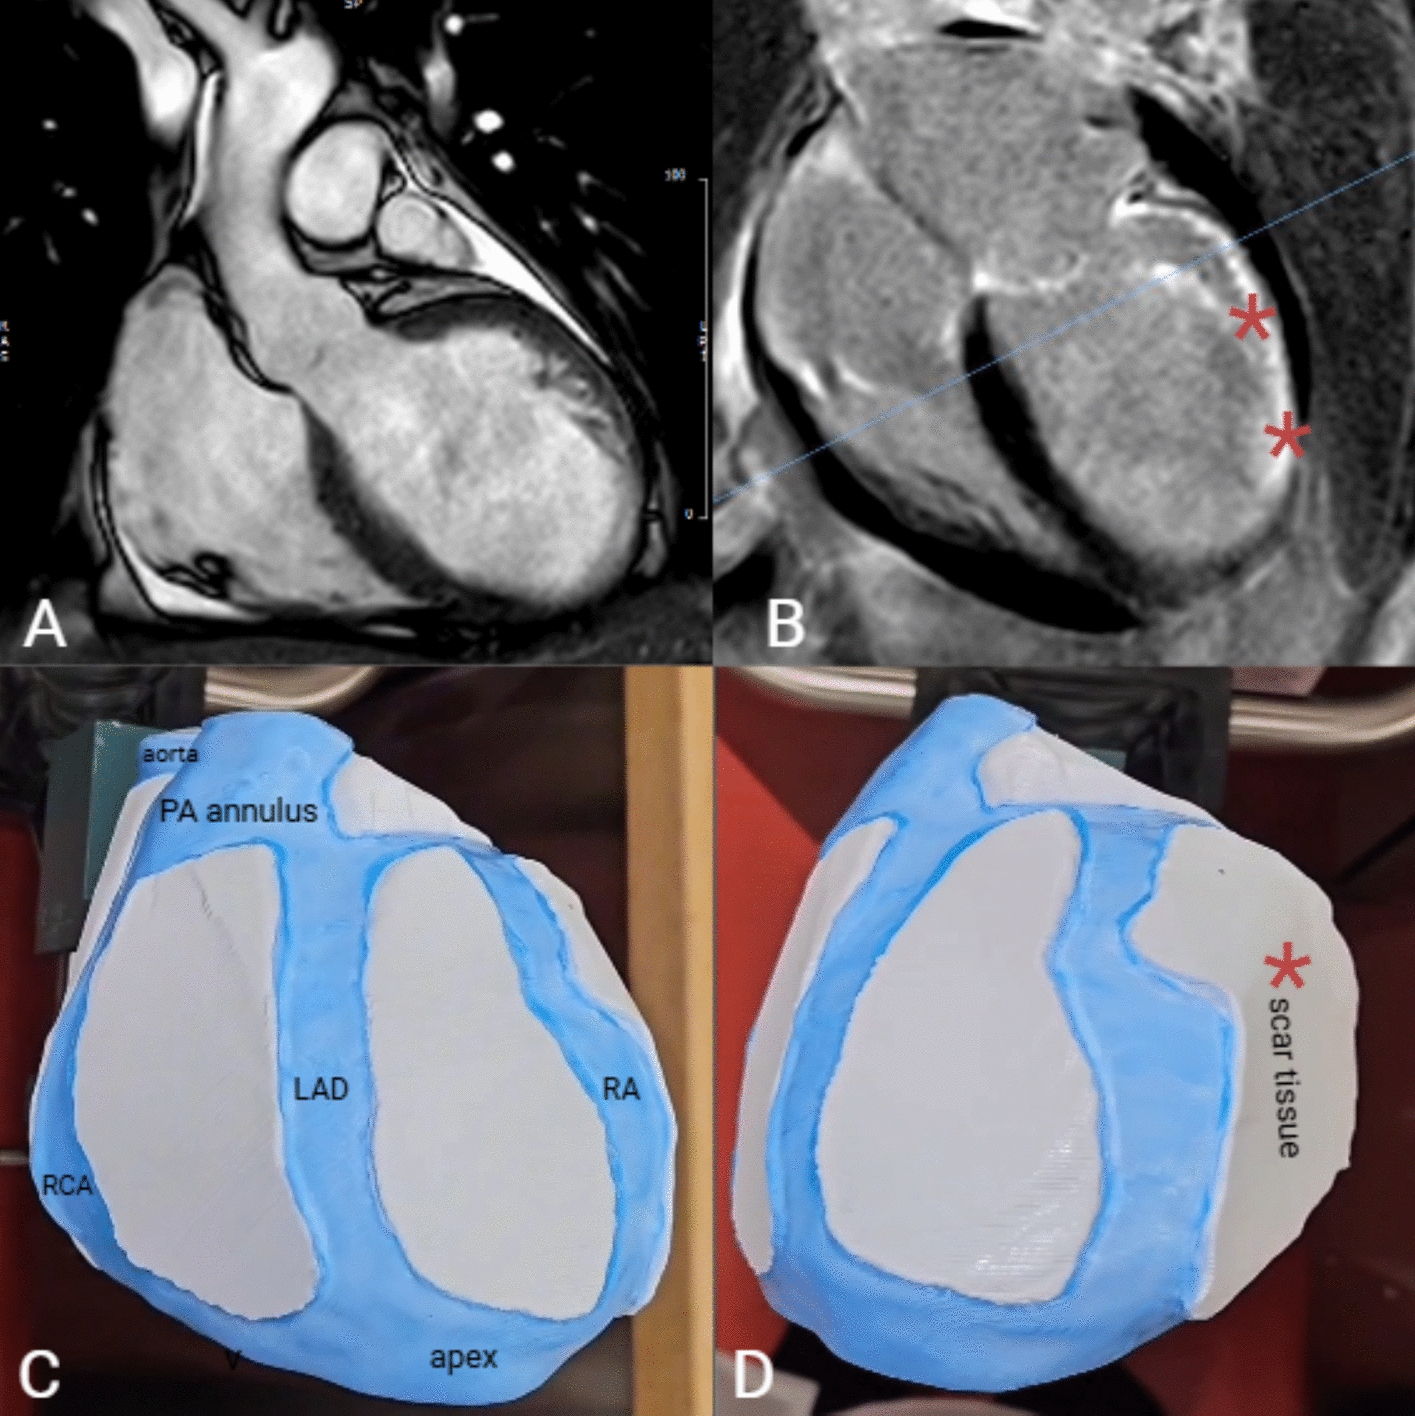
Late gadolinium enhancement cardiac MRI (A), with post-infarction scar indicated by red asterisks in (B). (C, D) 3D surgical guide showing anatomical landmarks: aorta, pulmonary artery (PA) annulus, and apex. Coronary protection designed for the right coronary artery (RCA), left anterior descending artery (LAD), and ramus angularis (RA)
